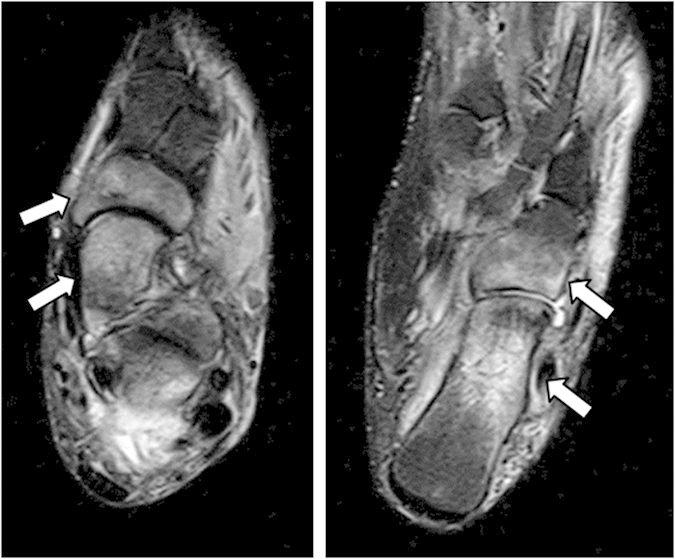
Frontal view STIR MR images. Although bone signal changes were broadly observed from the tarus to the talus. Right panel depicts signal changes in the calcaneus, cuboid. Left panel shows signal changes in the talus and navicular bones.
